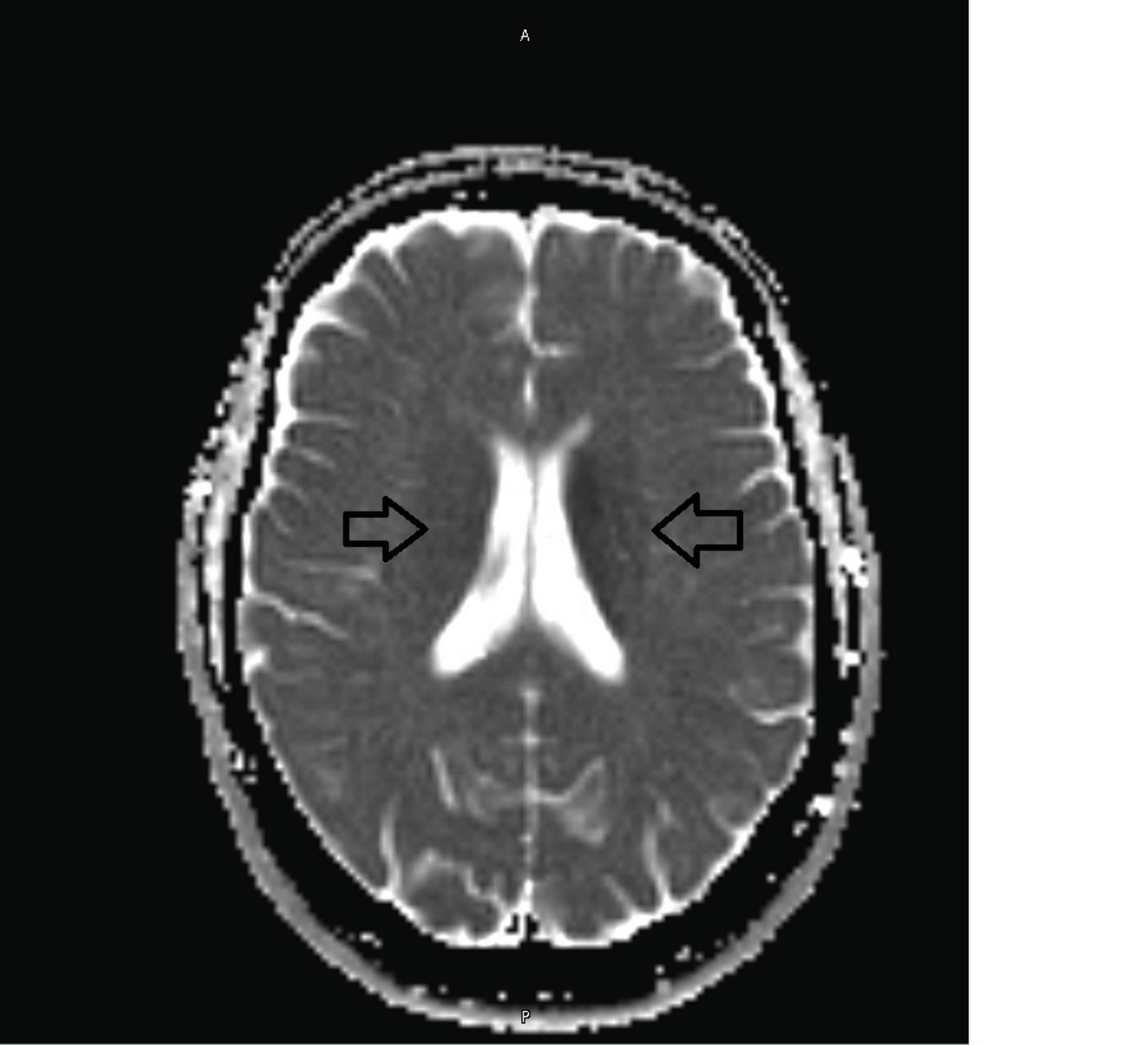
Magnetic Resonance Imaging (MRI) ADC image ADC: Apparent Diffusion Coefficient. Abnormal diffusion restriction in the basal ganglia and bilateral thalami, as described, is concerning; differential includes CJD, recent seizure activity and previous hypoxic/metabolic encephalopathy.
